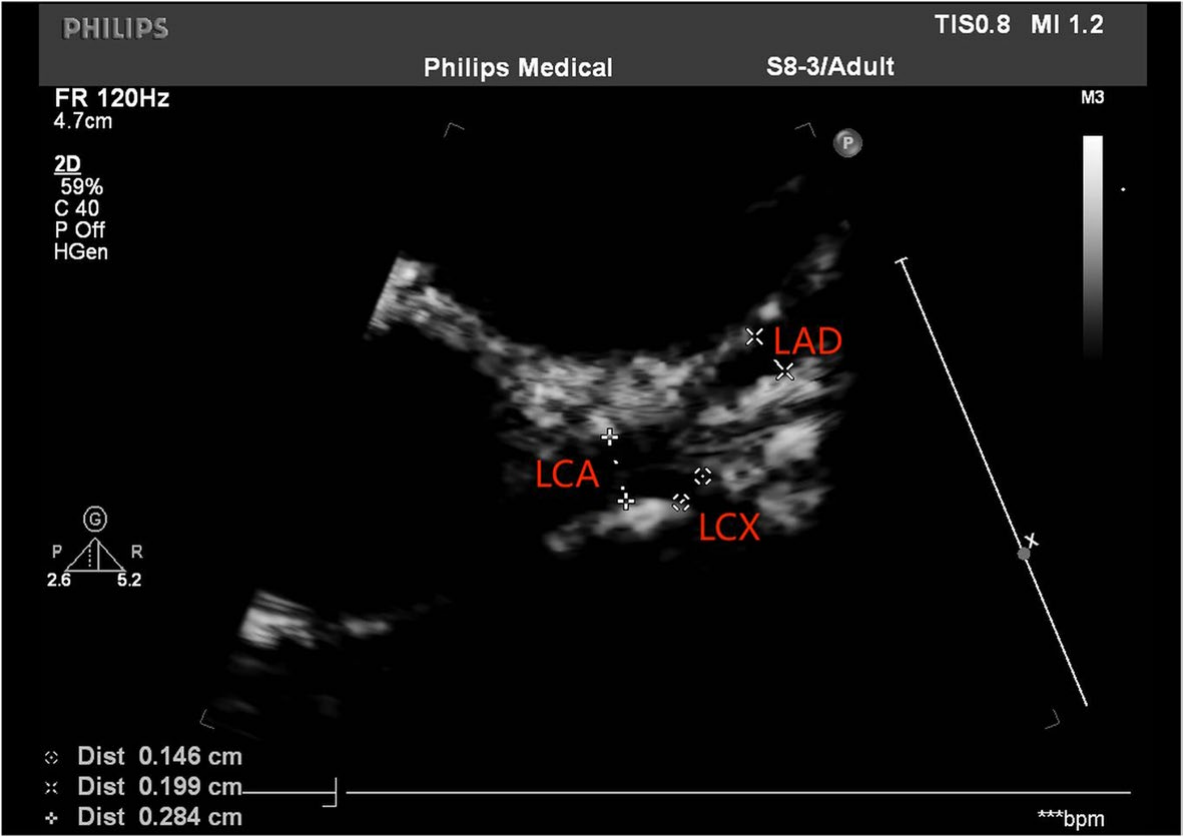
Ultrasound measurement of the left coronary artery and its branches
